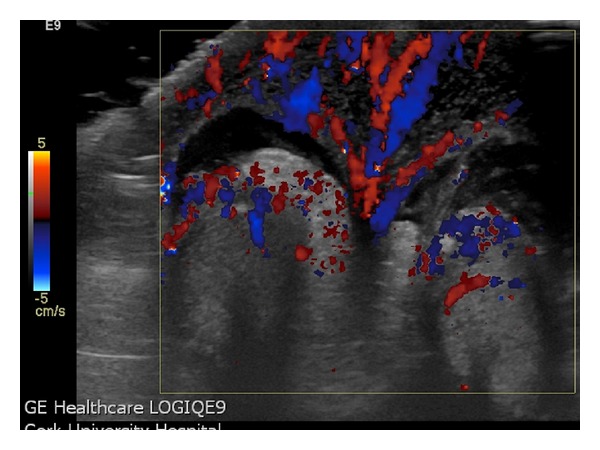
Transverse color Doppler image showing the “Fountain Sign” of increased blood flow in the edematous scrotal skin.
